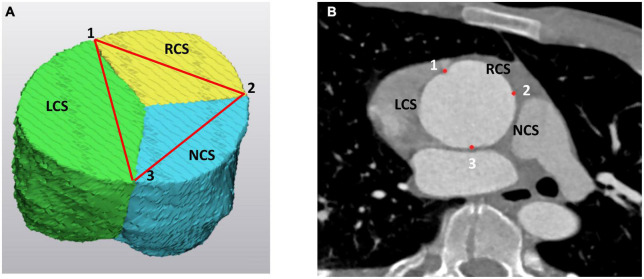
The three commissures at the level of the sinotubular junction (STJ) as (A) on the 3D model of the aortic root and (B) on the angio CT scan. The “*123*” landmarks correspond to the left (LCS), right (RCS), and non (NCS) coronary sinus commissures. On the 3D model the LCS is marked as green, the RCS is yellow and the NCS is blue. LCS, left coronary sinus; RCS, right coronary sinus; NCS, non-coronary sinus.
